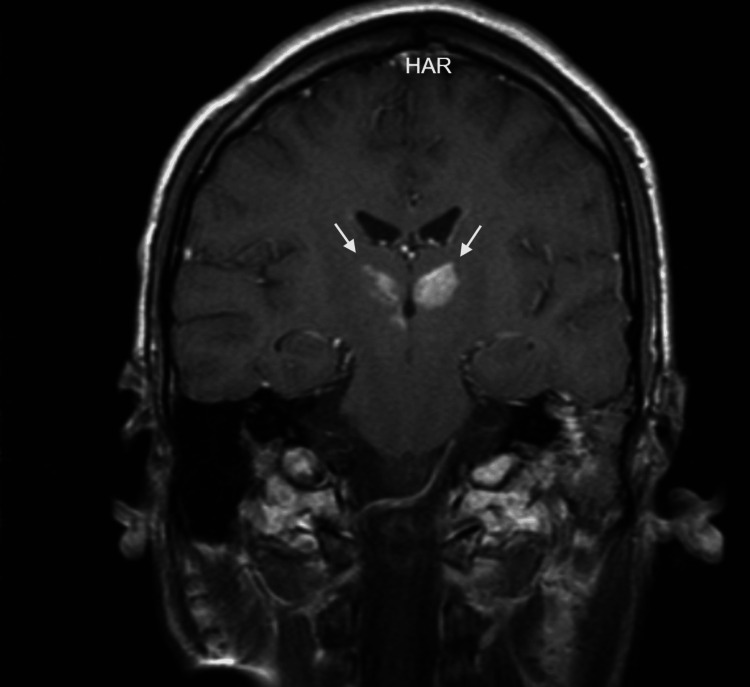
Coronal MRI of bilateral thalamic infarction secondary to occlusion of the artery of Percheron Coronal T1-weighted images were also acquired following the administration of gadolinium contrast. HAR: Holographic augmented reality.
